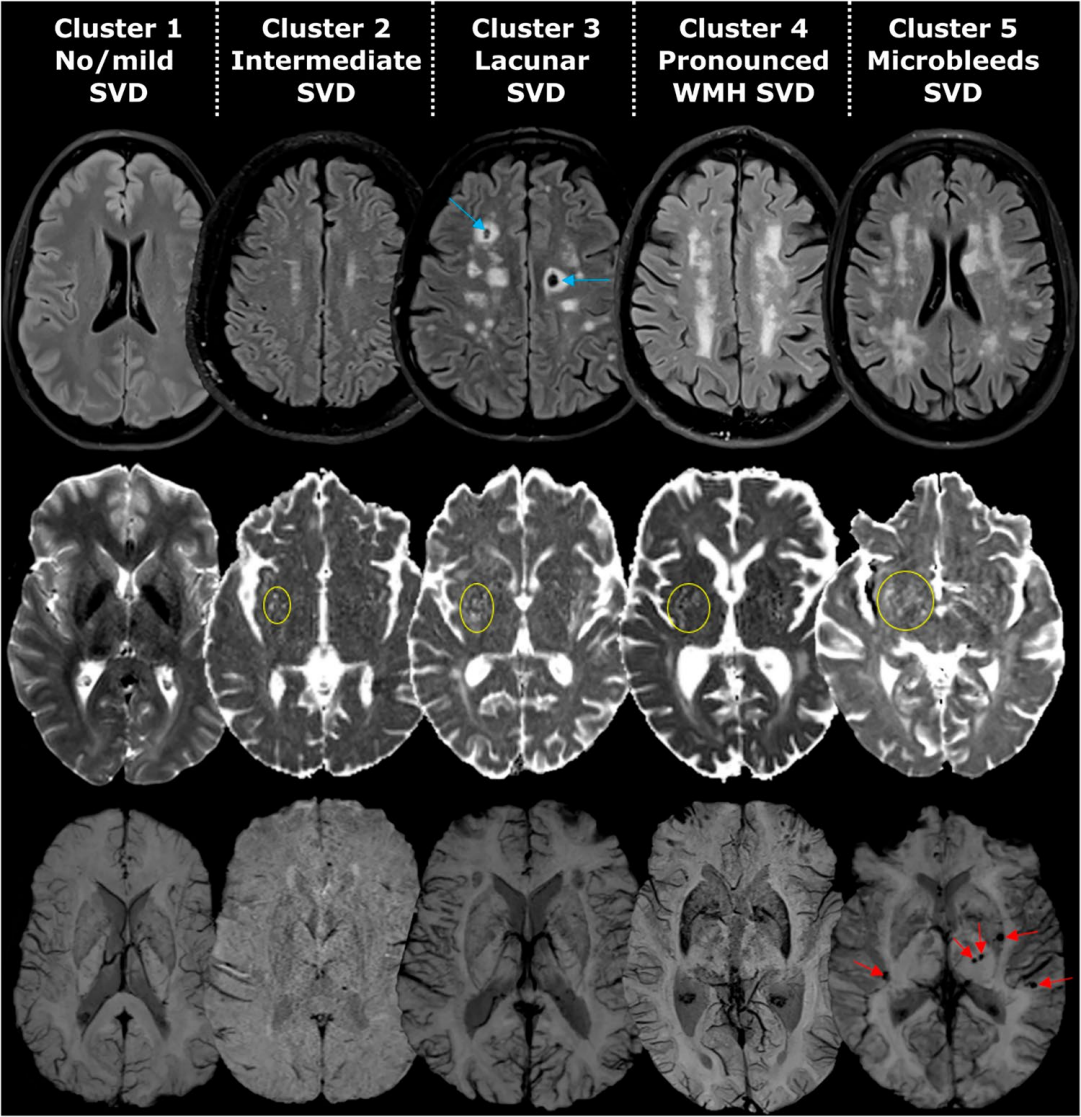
Example MRIs for each cluster. T2 FLAIR, DWI b0, and susceptibility-weighted MRI of example patients in each cluster. Coloured markings highlight the relevant SVD features (blue lacunes, yellow EPVS, red microbleeds)
